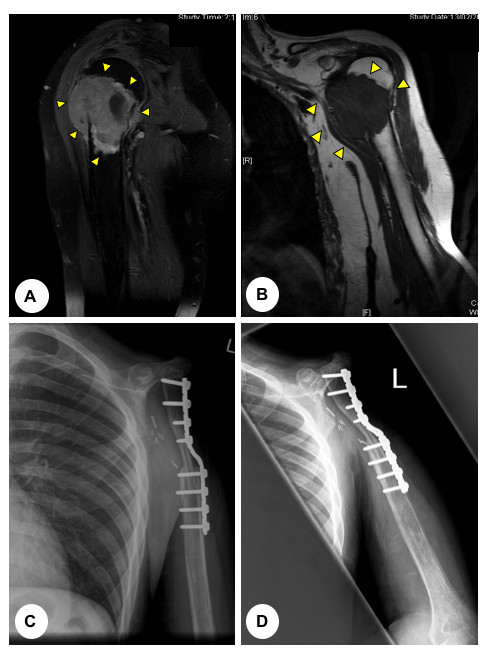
(A) T2-weighted and (B) T1-weighted coronal magnetic resonance images (MRI) of a 43 year old man presenting with osteosarcoma involving the proximal humerus (arrows). (C) Antero-posterior and (D) lateral post-operative plain radiographs demonstrating the surgical management, which comprised of a proximal humeral resection and a claviculo-prohumeral reconstruction of the shoulder.
